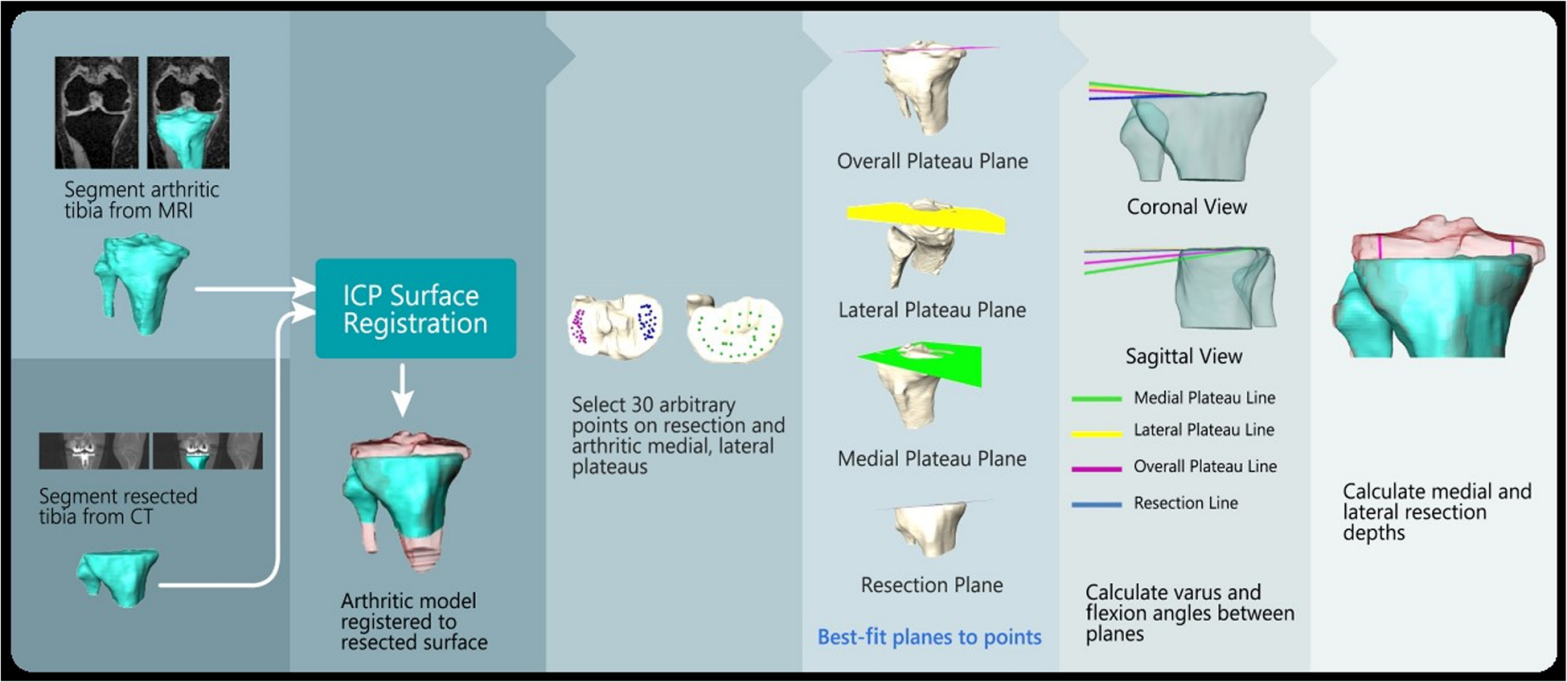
Registration process of surface models, point selection, plane fitting, and calculations. [The registration process for the various steps are represented. Panels one and two represent pre-operative MRI and post-operative resected CT surface models; panels three and four represent point selection and plane fitting, respectively; panels five and six represent calculations.]
